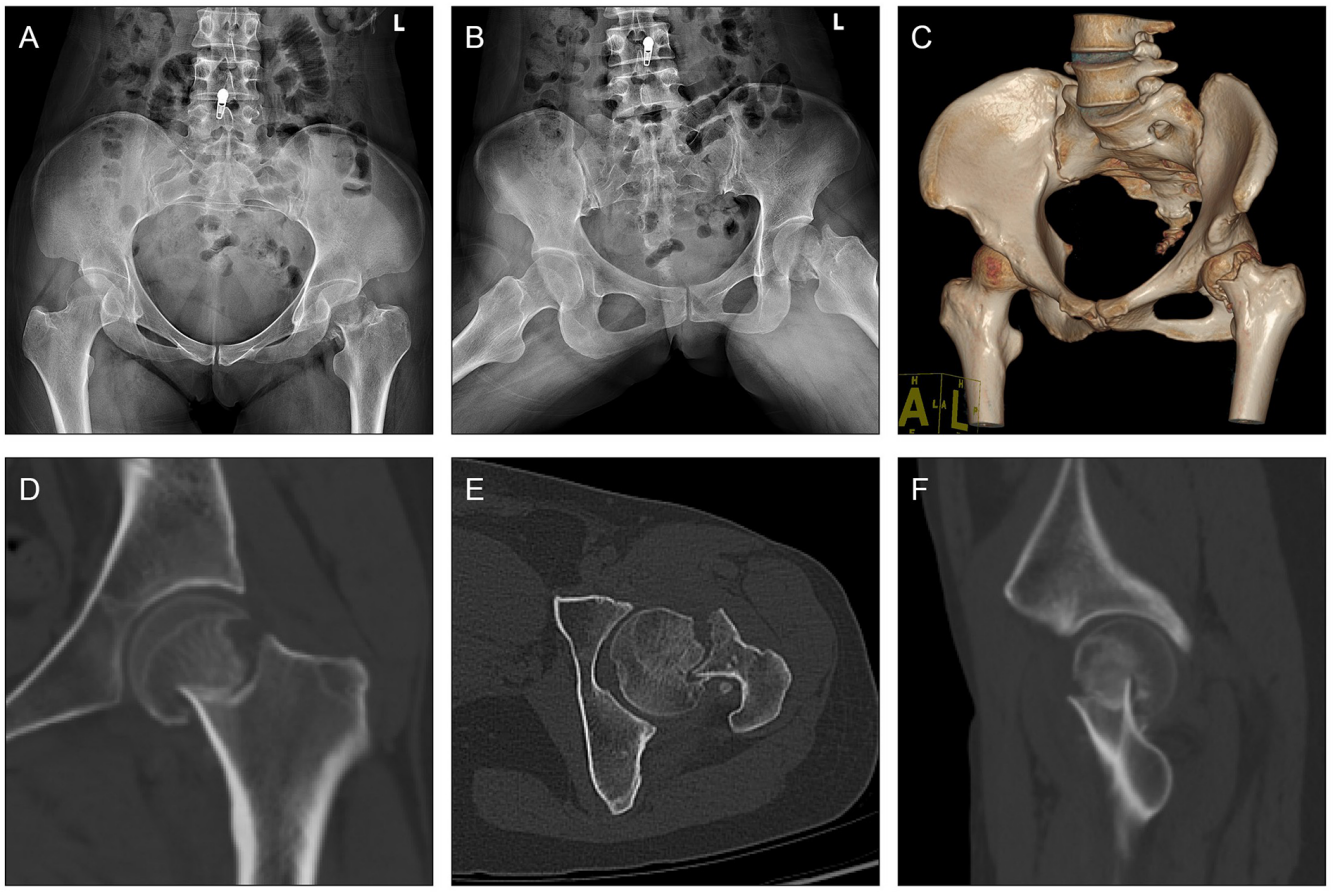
Case presentation. (A,B) Pre-operative x-rays and (C–F) CT scan of a 36-year-old female who was diagnosed with displaced subcapital femoral neck fracture (AO/OTA classification: 31B1.3, Pauwels classification: Type I) caused by a fall from flat during a sport accident.
